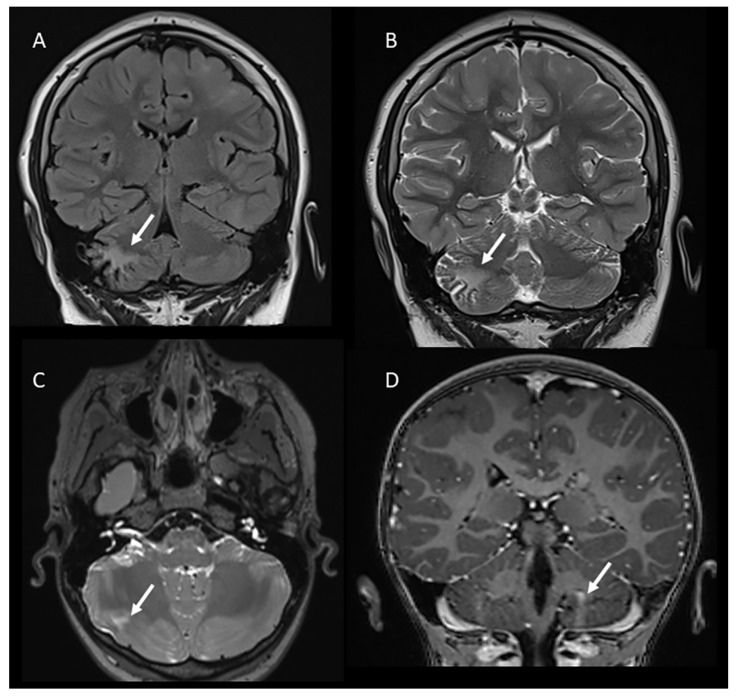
Coronal FLAIR (A) and T2-weighted (B) images demonstrating a cerebellar tuber in the right hemisphere in a 13-year-old male. Axial T2-weighted (C) and coronal post-contrast T1-weighted images (D) showing other examples of cerebellar tubers (arrows) in two patients with tuberous sclerosis (8 and 5 years old, respectively).
